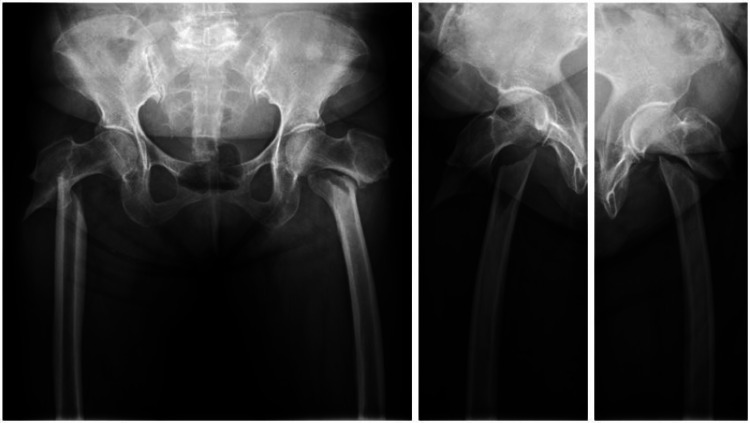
Anteroposterior and lateral radiographs of hip joint of a 66-year-old female with bilateral subtrochanteric complete atypical femoral fracture.
